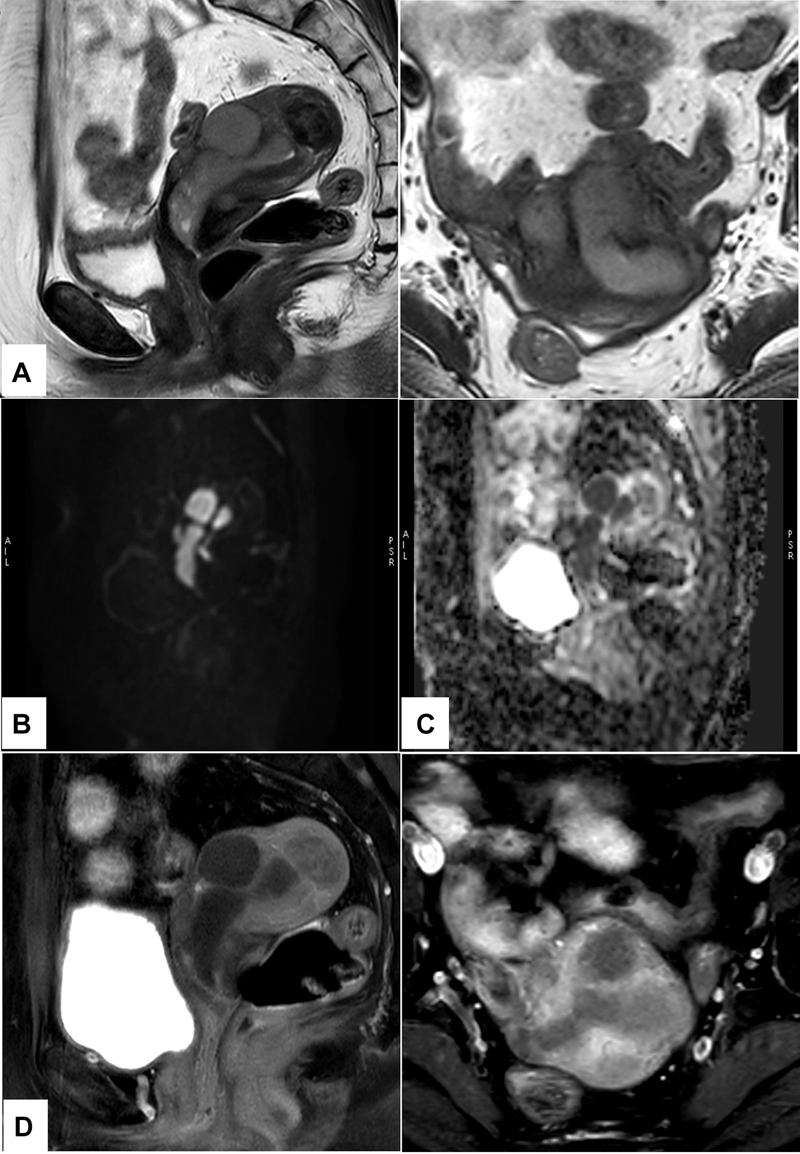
Magnetic resonance imaging (MRI) pelvis showing linear polypoidal lesion within the endometrial cavity. It appears hyperintense on T2-weighted (T2W) sagittal and axial images (
